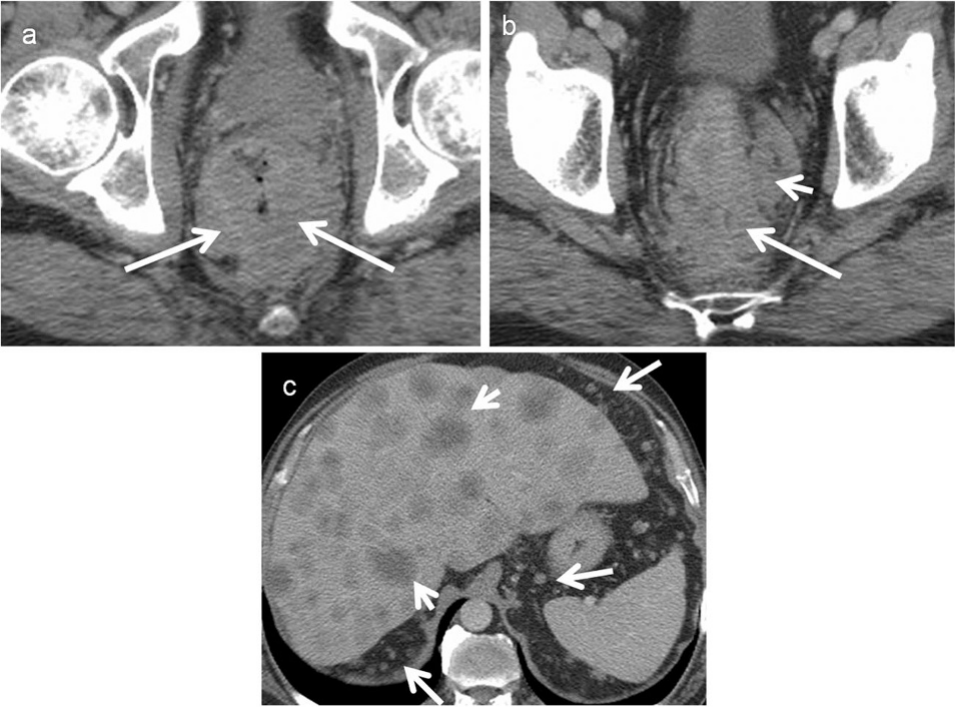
Rectal carcinoid in a 66-year-old woman. a, b Axial contrast-enhanced CT reveals lobulated circumferential thickening of the rectal wall (*long arrow*) with perirectal lymph nodes (*short arrow*). c Axial contrast-enhanced CT show extensive liver (*short arrow*) and peritoneal (*long arrow*) metastases
